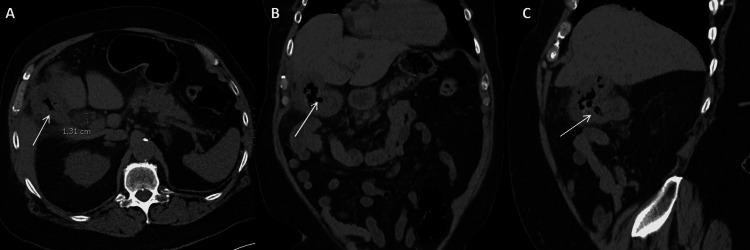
Second Preoperative CT This is the second preoperative CT and was completed six weeks after the first preoperative CT. The white arrows identify the cholecystocolonic fistula. Pane A shows the transverse section with the common bile duct measuring 1.31 cm. Pane B shows the coronal section. Pane C shows the sagittal section.
